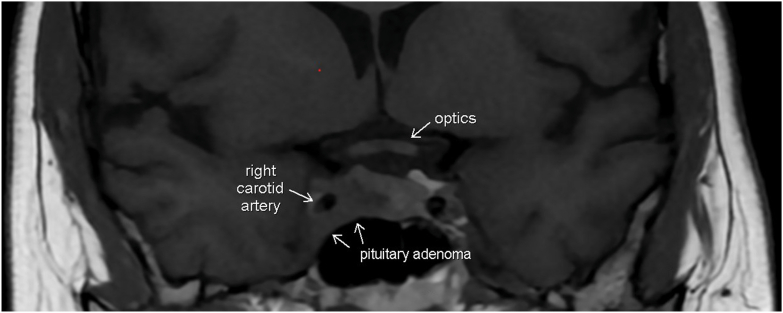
MRI pituitary coronal image revealing sellar mass with invasion of the *right* cavernous sinus; extension around the *right* internal carotid artery.
